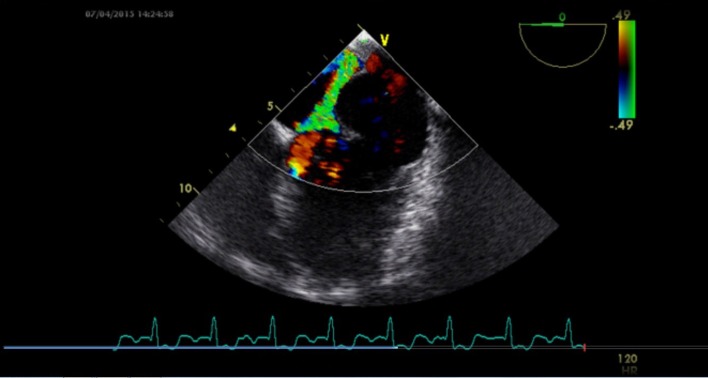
Transesophageal echo in four-chamber view with transducer positioned in esophagus displaying severe mitral regurgitation with an eccentric jet.
